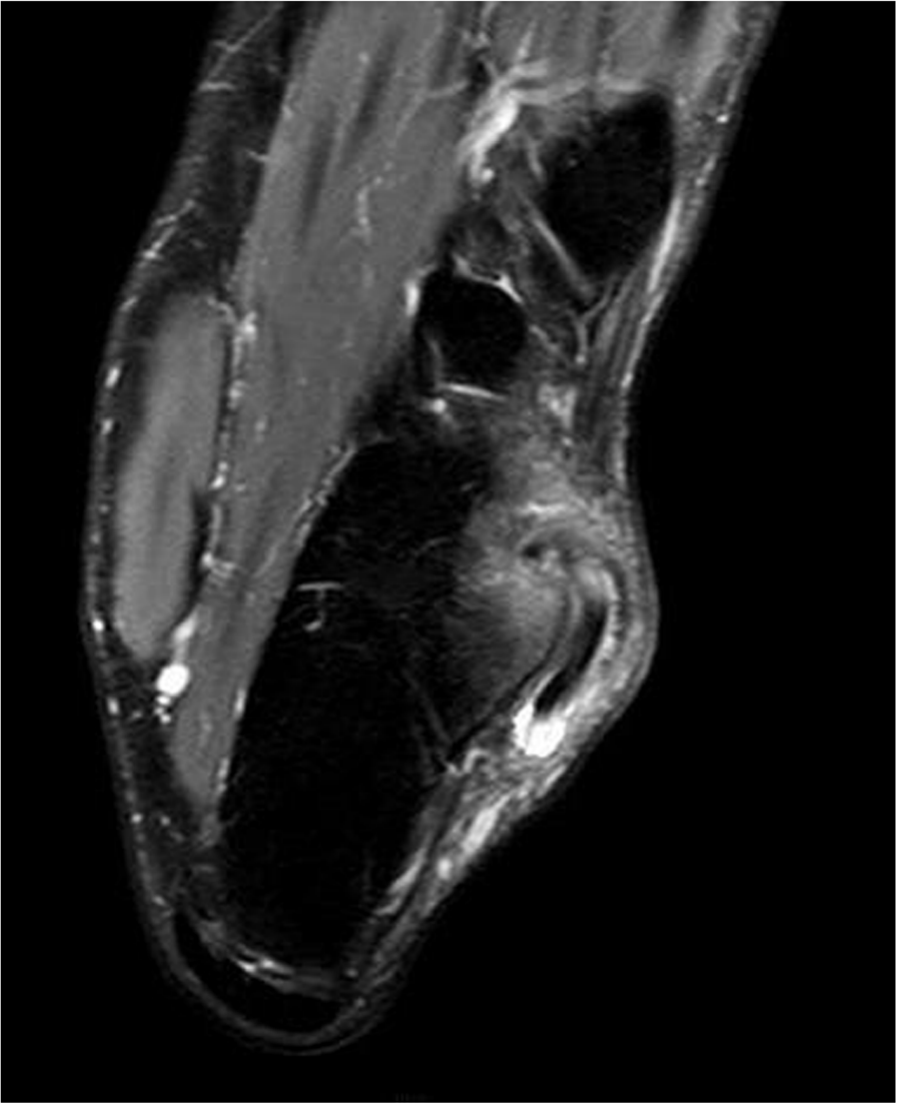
Axial MRI section of the enlarged and dislocated peroneus longus tendon over the peroneal tubercle. Intensive subchondral bone oedema of the peroneal tubercle
